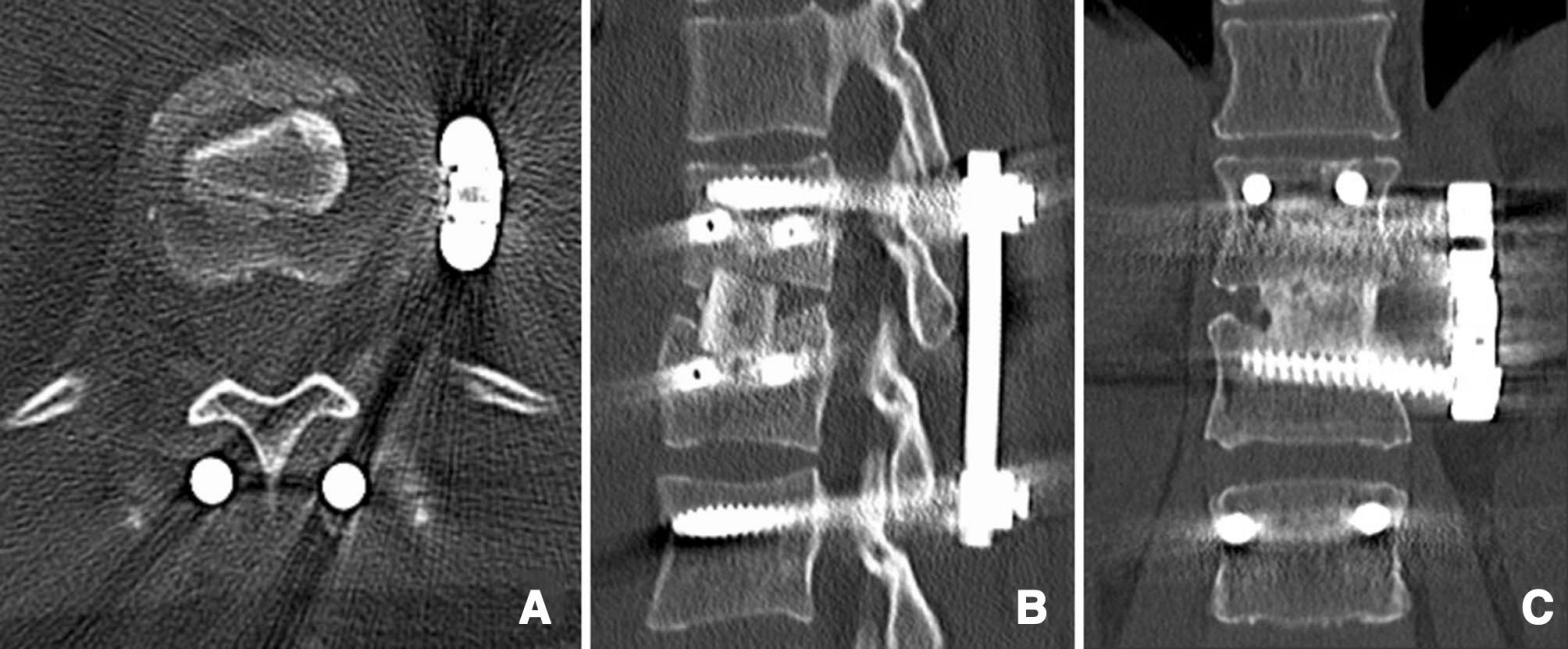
Postoperative CT of a 38-year-old woman with a superior burst-split fracture (Magerl et al.^19^ A3.2.1, AOSpine A4^1^) of L1 treated by short segment posterior instrumentation and anterior one-level spondylodesis using an iliac crest bone graft (ICBG). Representative axial (A), sagittal (B) and coronal (C) slices.
